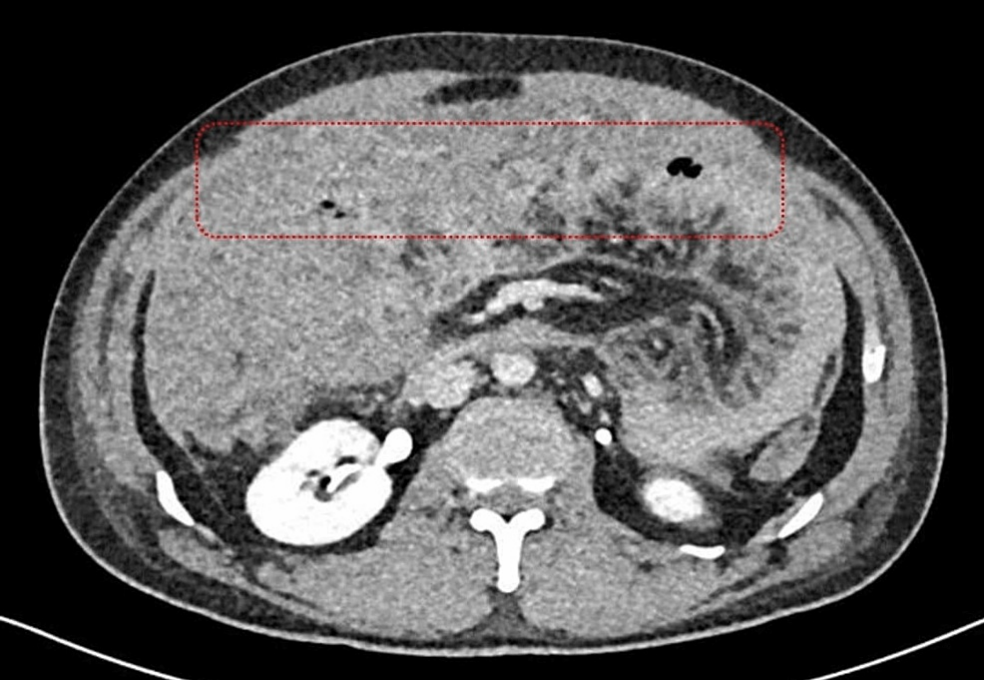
Computed tomography scan showing diffuse thickening of the omentum (omental caking)
